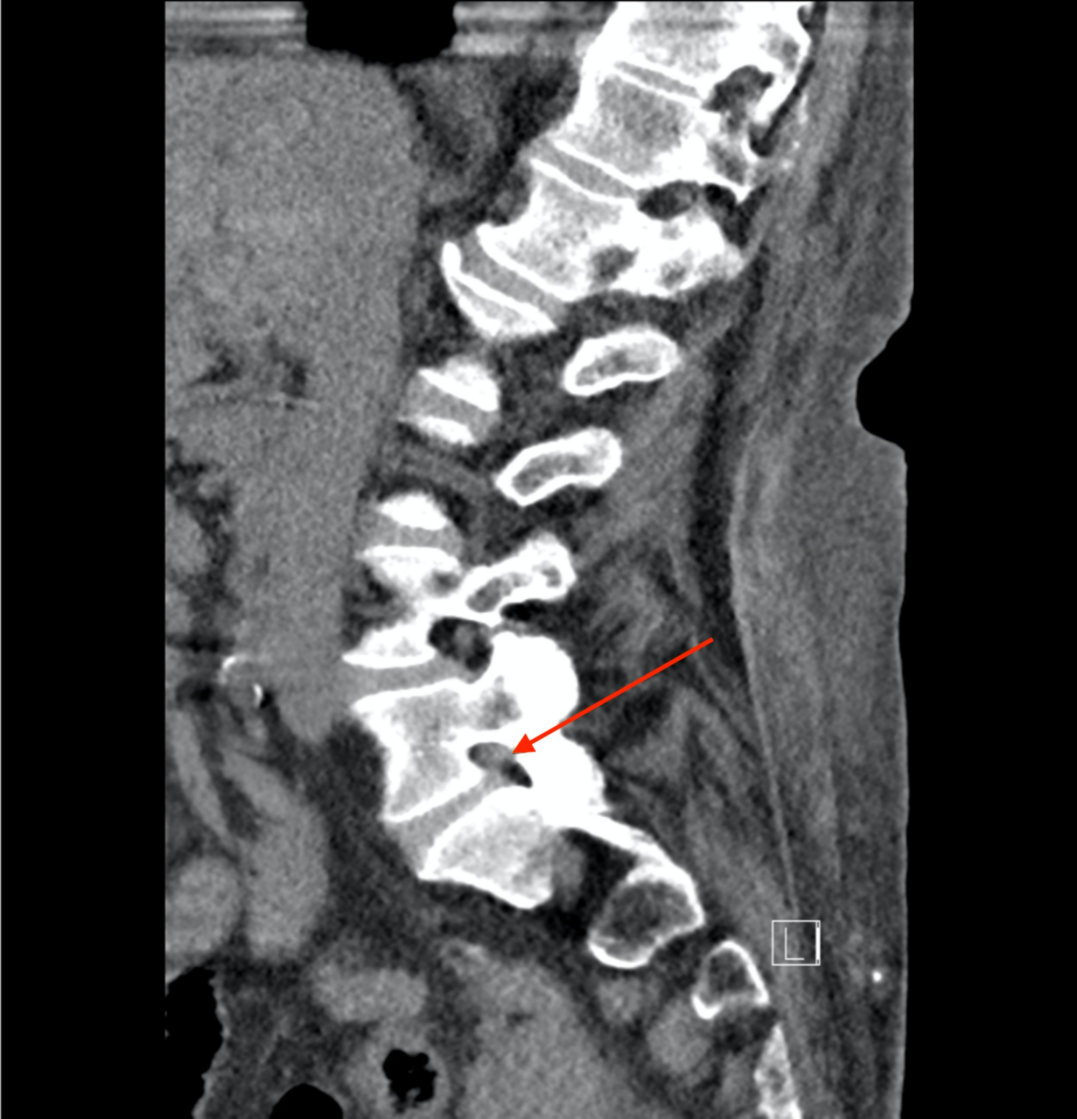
Lumbar CT without contrast The red arrow points to neuroforaminal narrowing at L5-S1 on the right
